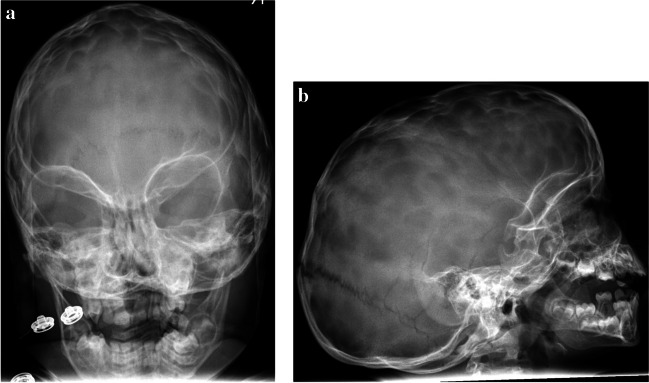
a Skull X-ray of patient number 7, frontal view. b Skull X-ray of patient number 7, lateral view
